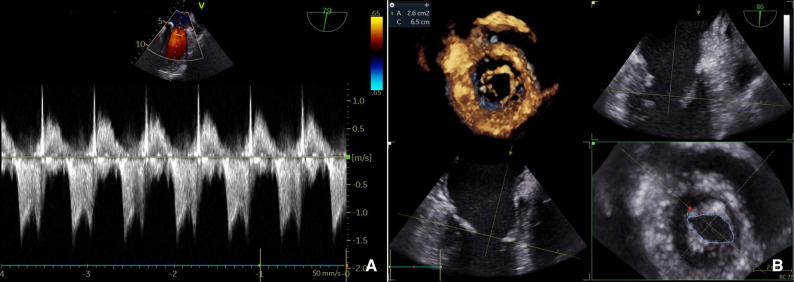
Post-procedural transesophageal echocardiogram. Significant improvement in mitral valve opening. A Maximum velocity: 1.5 m/s, peak gradient: 9 mmHg, mean gradient: 3.8 mmHg. B Mitral valve area by 3D planimetry: 2.6 cm²
